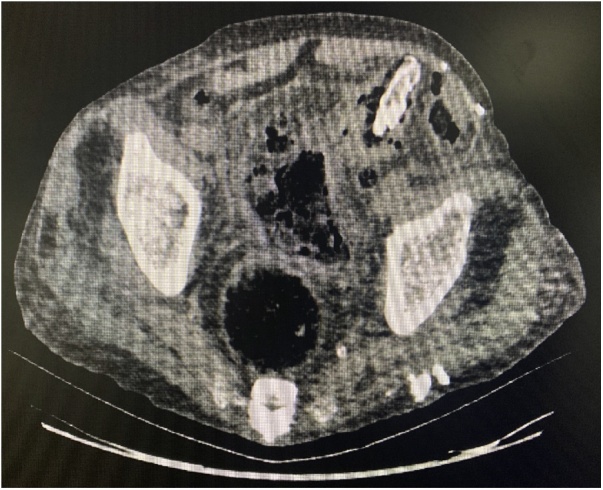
Abdominal CT scan with neumoperitoneum with a strange body into de left colon lumen.
